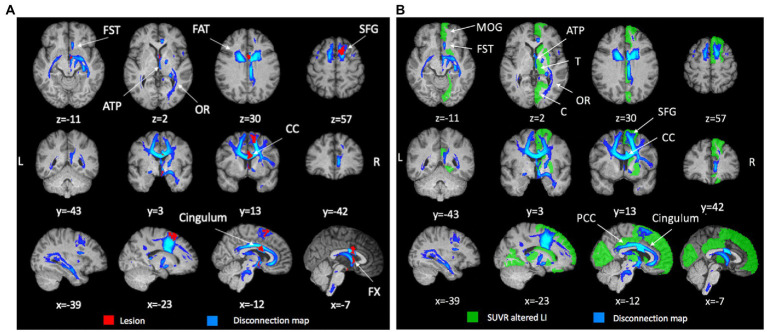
Anatomical lesion, structural disconnection map and metabolic asymmetry. (A) Anatomical lesion and associated structural disconnection map. T1-weighted structural MRI scan showing the anatomical lesion (red) in the midline frontal structures and the associated structural disconnection (blue) extending posteriorly and contralaterally (FST, fronto-striatal tract; ATP, anterior thalamic projection; OR, optic radiation; FAT, fronto-aslant tract; SFG, superior frontal gyrus; CC, corpus callosum). (B)
